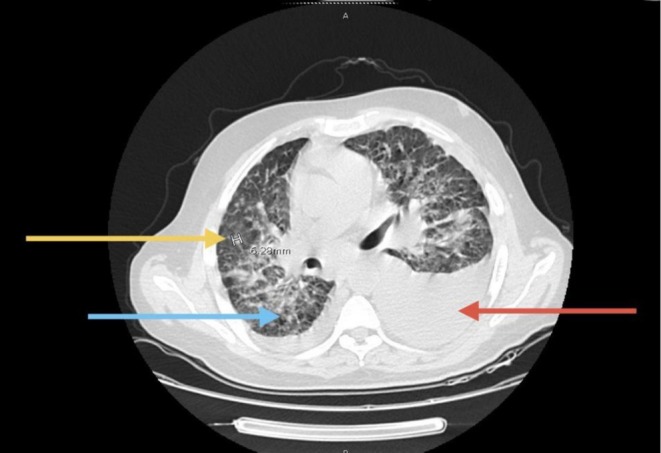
Pulmonary involvement and pleural effusion. Chest CT showing mild right‐sided and moderate left‐sided pleural effusions (red arrow), associated with bilateral ground‐glass opacities, patchy consolidation, interstitial lung thickening (blue arrow), and prominent secondary pulmonary nodules at the lung bases (yellow arrow), consistent with pulmonary dissemination of the disease.
